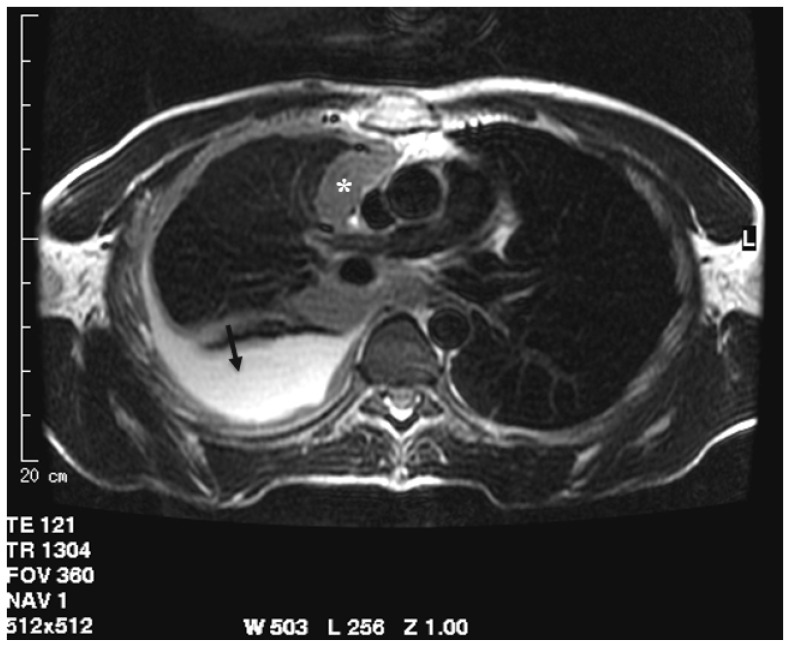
T2-weighted image acquired in the axial plane clearly demonstrates circumferential pleural thickening with mediastinal pleura involvement (asterisk) and pleural effusion (arrow).
